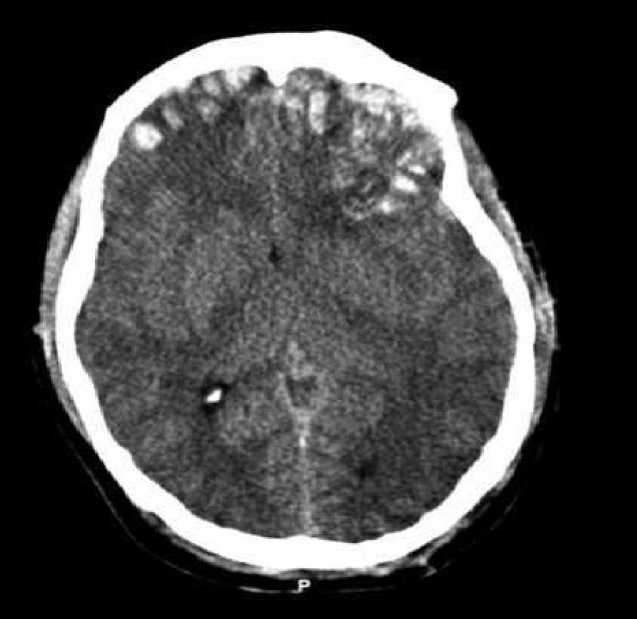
(Initial CT.tif) – Preoperative CT scan of patient while he had a GCS of 14.
